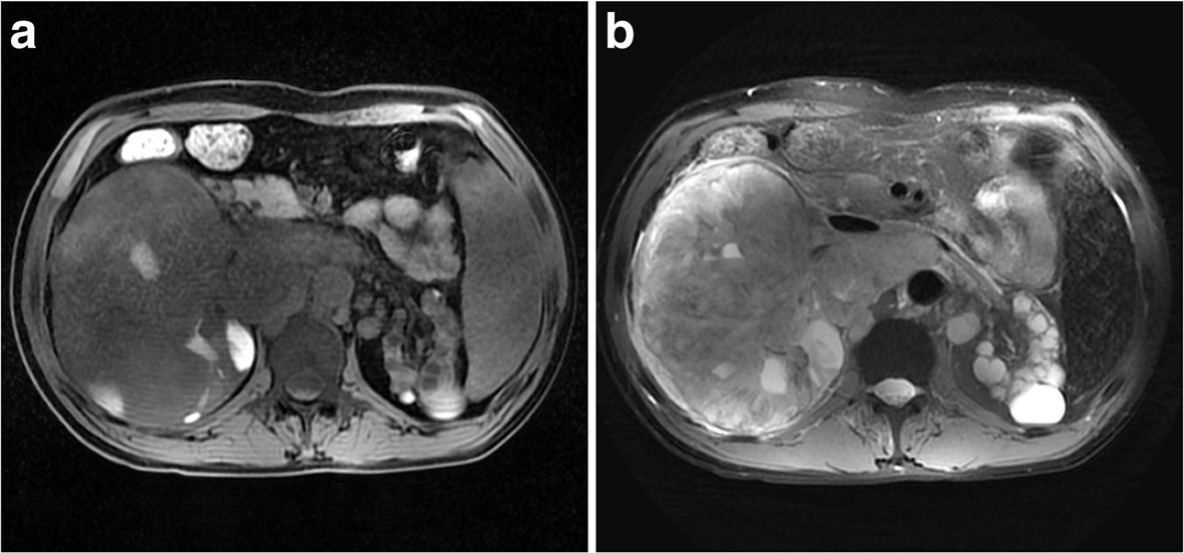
Magnetic Resonance Image (MRI) of the abdomen in a 40-year-old man with Xp11.2 translocation RCC. a, b, Axial T2WI (a) and plain T1WI (b) showed a large, well-defined, irregular mass (T2, high-low heterogeneous signal intensity; T1, iso-signal intensity) with patchy hemorrhage and necrosis in the mass and enlargement of abdominal lymph nodes
